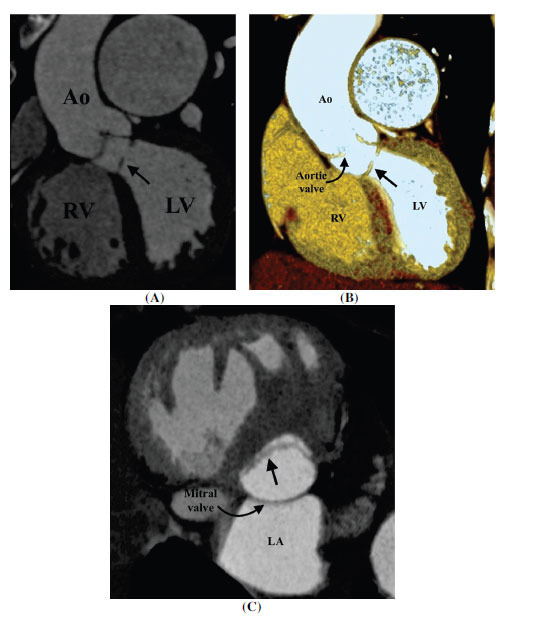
Membrane-type subaortic stenosis. (A) Coronal contrast-enhanced computed tomographic angiography image and (B) thin volume rendered technique image showing a shelf-like fibromuscular in the subaortic region (arrow). (C) Axial minimum intensity projection image showing the classical appearance of the subaortic membrane (arrow). It falls short in the aortomitral region forming a horseshoe shape.
